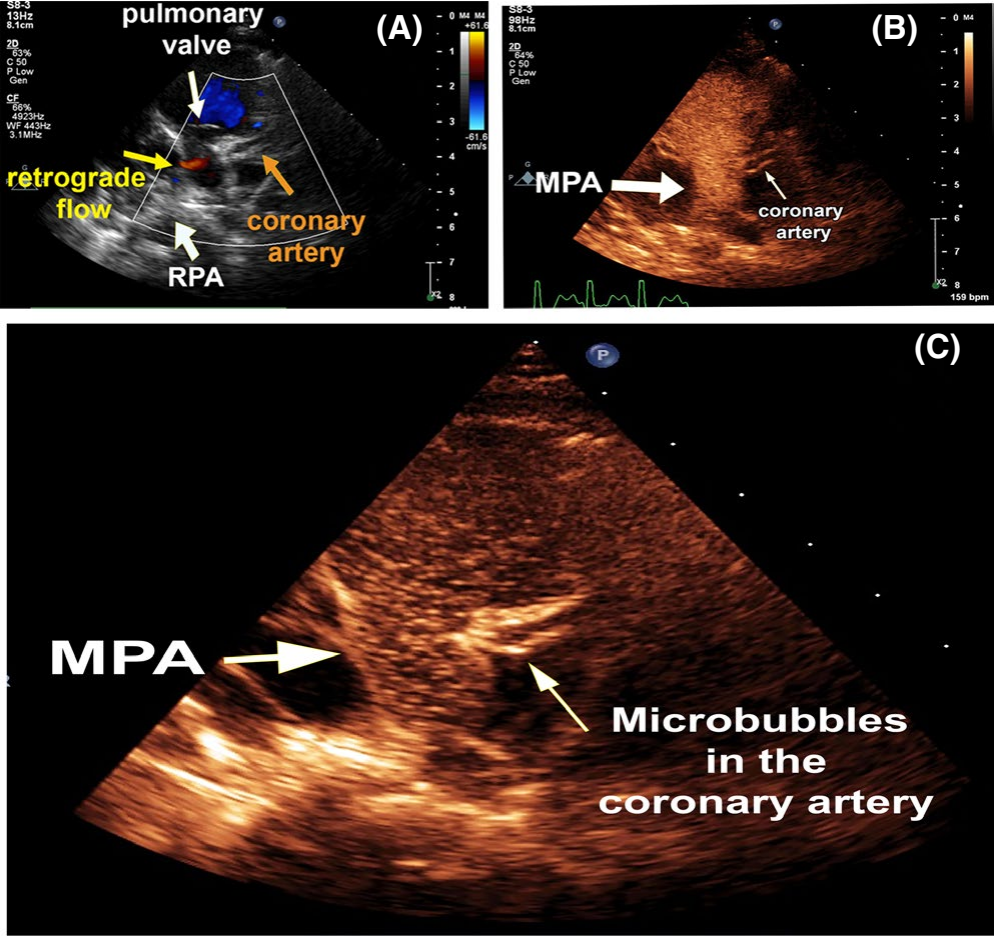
Transthoracic two‐dimensional echocardiography. A, parasternal short‐axis view shows pulmonary valve (white arrow), main pulmonary artery, some segments of the anomalous left coronary artery (orange arrow), and the retrograde flow from the orifice of the anomalous left coronary artery (red flow) characterized by the yellow arrow. B, parasternal short‐axis view, with turning on of the color map for better visualization, indicates the main pulmonary artery is filled with microbubbles of the agitated normal saline. C, microbubbles are seen within the lumen of the anomalous left coronary artery. RPA = right pulmonary artery, MPA = main pulmonary artery
